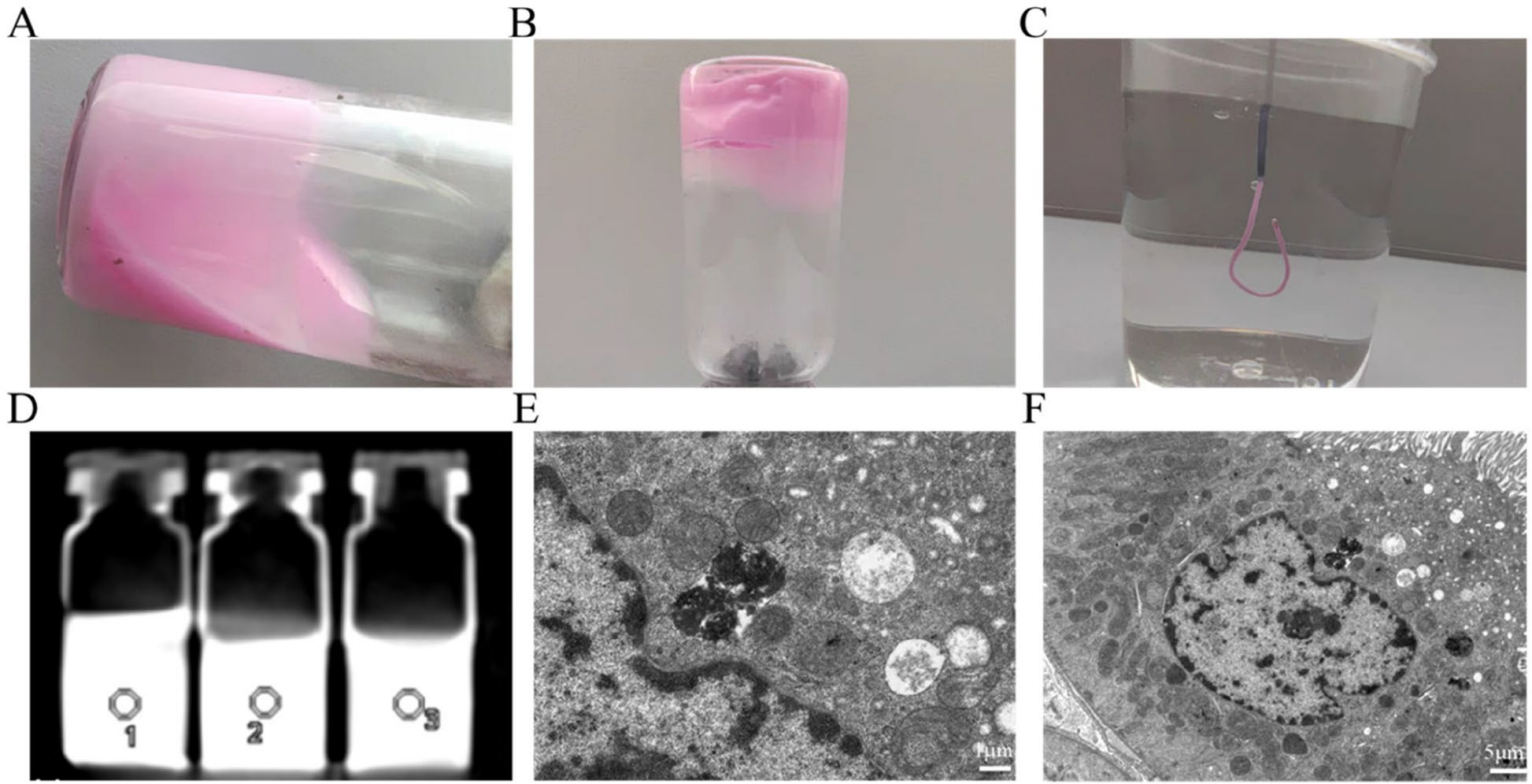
Properties of PGEL. A PGEL is a flowable solution at low temperature and B becomes a solid gel at high temperature (> 36 °C). C PGEL is injected into 37 °C water through a 2.7 F microcatheter and rapidly solidifies as a gel. D Comparison of X-ray attenuation of PGEL with Iomeprol and Omnipaque (from left to right). E, F TEM images of renal artery embolization with PGEL at different scales. PGEL is seen in the renal arteries and glomeruli (black particles are PGEL).
